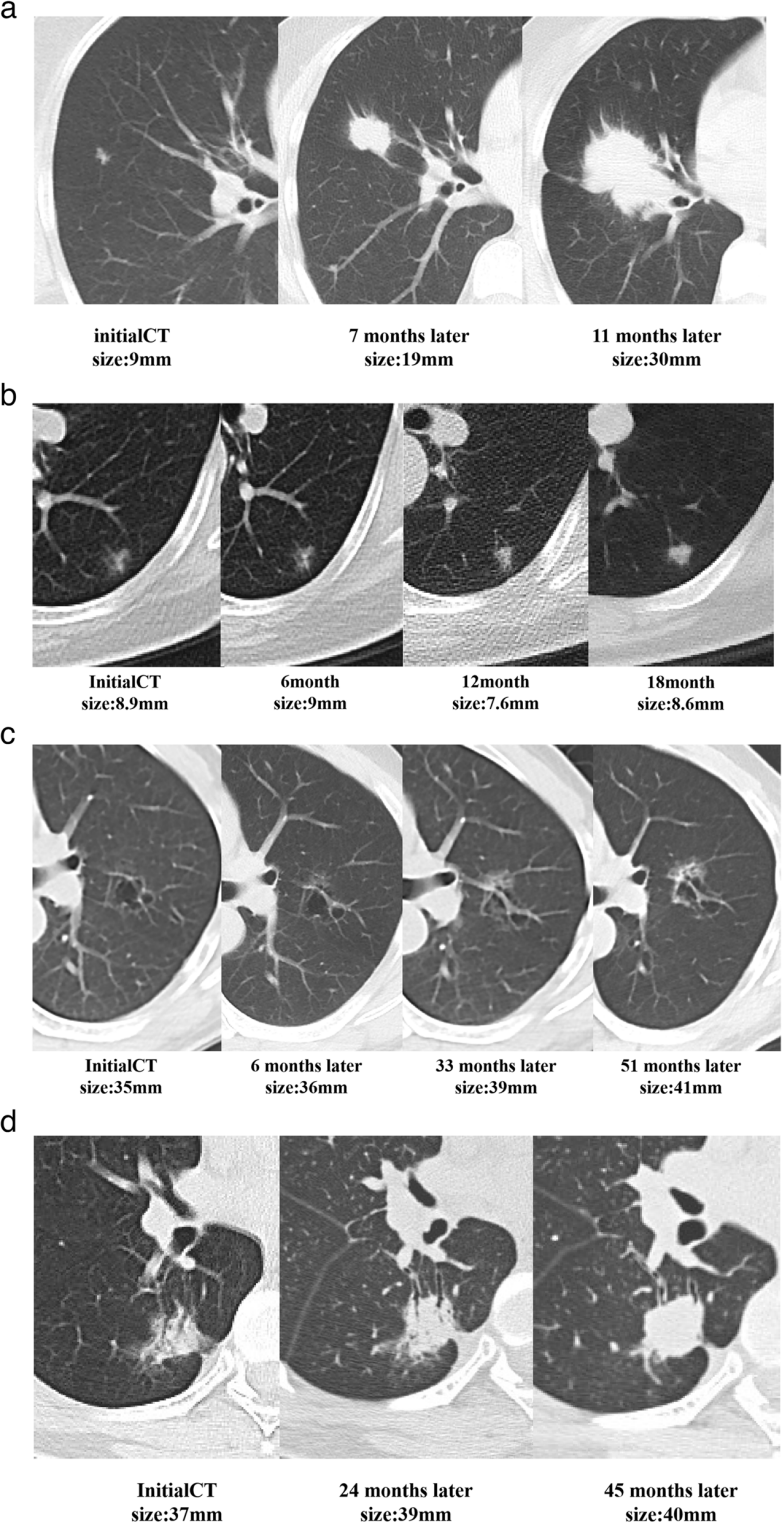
Axial CT images show the evolution of type I-IV SLADCs during follow-ups. a Sequential images in a 47-year-old woman who had LADC show that the tumor enlarged over time with lobulation, spiculation, and pleural retraction appeared. b Sequential images in a 60-year-old woman who had LADC show that the tumor was stable firstly, then decreased with solid components and density increased, and then increased again. c Sequential images in a 65-year-old man who had LADC show that the cystic airspace enlarged, cystic walls thickened, and wall nodules appeared. d Sequential images in a 58-year-old woman who had LADC show that a focal consolidation enlarged over time, with attenuation increased as well as air bronchogram and GGO component gradually disappeared, and eventually became a solid mass
